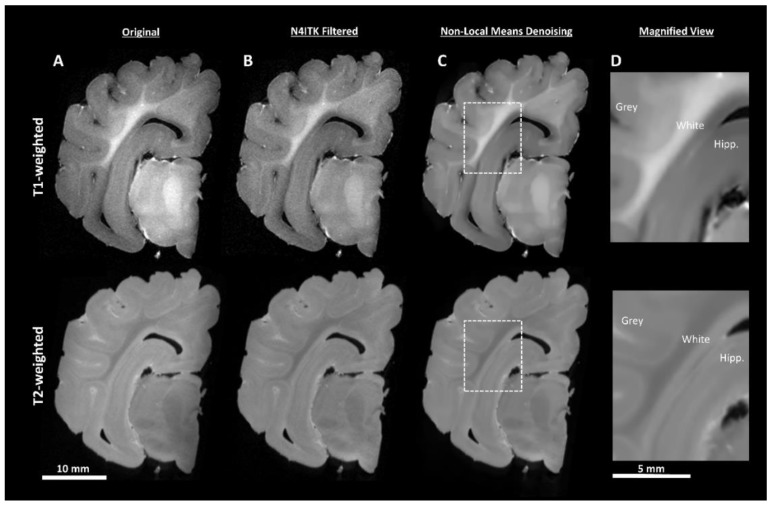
Image pre-processing demonstrated by T1- and T2-weighted coronal sections (A). N4ITK bias field correction (B) removes bright and dark patches in the image resulting in more uniform tissue-type intensity. The use of non-local means denoising (C) attenuates the high-frequency noise in the image, creating more uniform tissue boundaries. A magnified view of the boxed regions is shown in (D) encapsulating part of the cortex (White and Grey) and hippocampus (Hipp.) All images within each have normalized histograms to allow for quality comparisons.
